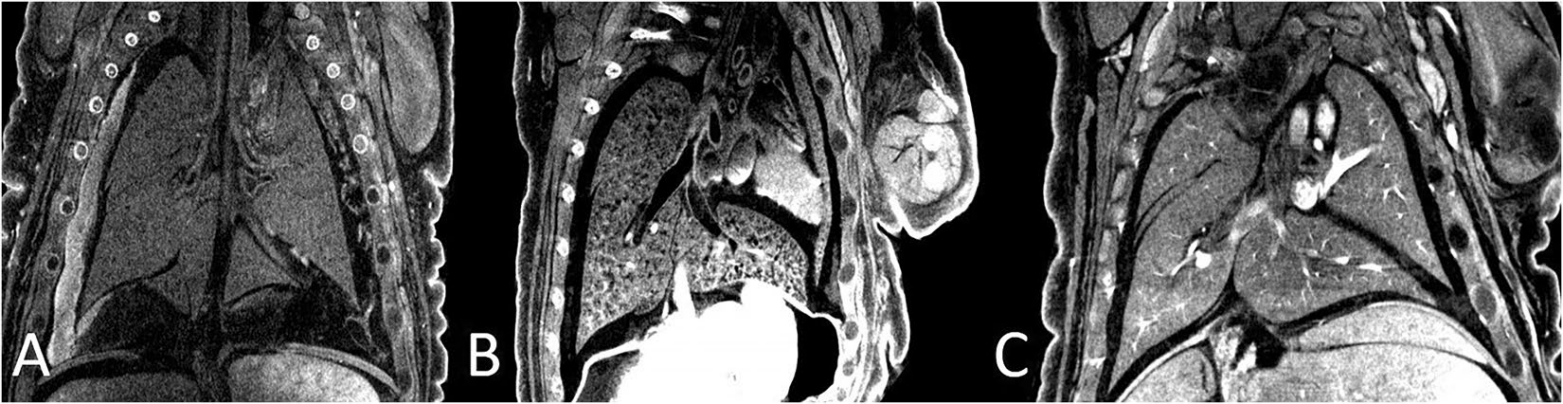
Micro CT images of the fetuses. (A) Injection of the contrast directly into the right ventricle; (B) Upper half body of the fetus embedded into the Lugol solution; (C) Partial peeling of the skin embedded in Lugol solution.
